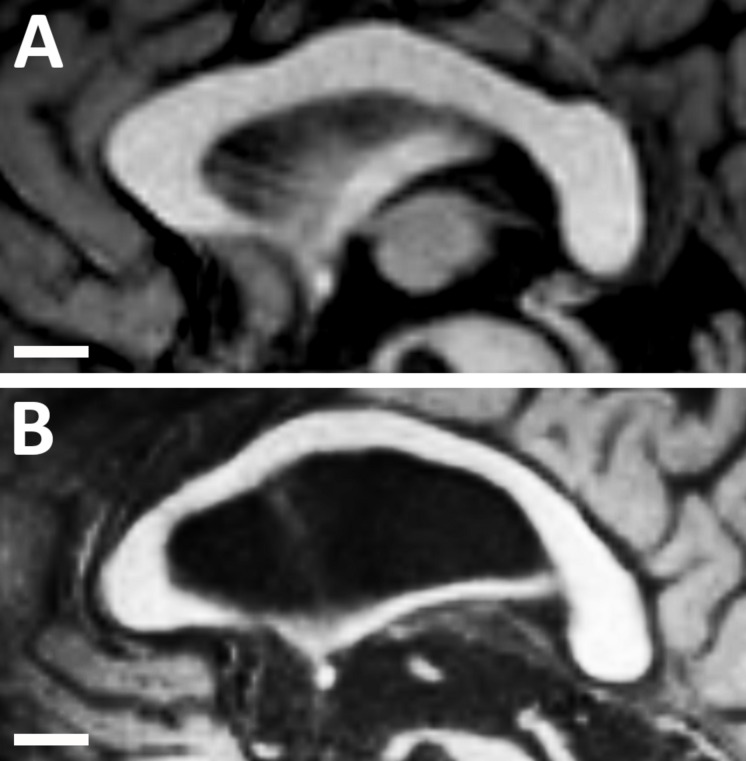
Midsagittal magnetic resonance images of the corpus callosum and lateral ventricle in (A) a healthy younger adult (aged 30 years); and (B) a healthy older adult (aged 68 years). Note the structural changes with age as the lateral ventricle becomes much larger and the corpus callosum becomes much thinner. However, the length of the corpus callosum remains approximately constant.
